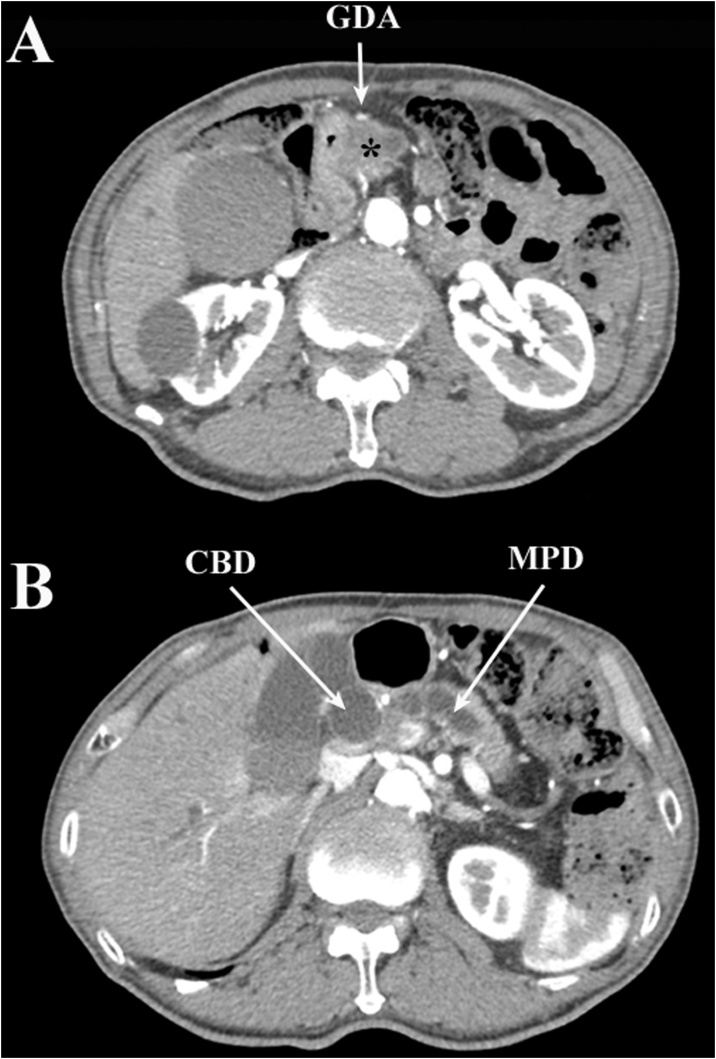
Scans of early-phase preoperative computed tomography with contrast medium; A: the gastroduodenal artery passing over the surface of the pancreatic head without tumor invasion; the asterisk indicates the pancreatic head cancer; B: the common bile duct and main pancreatic duct are considerably dilated.
